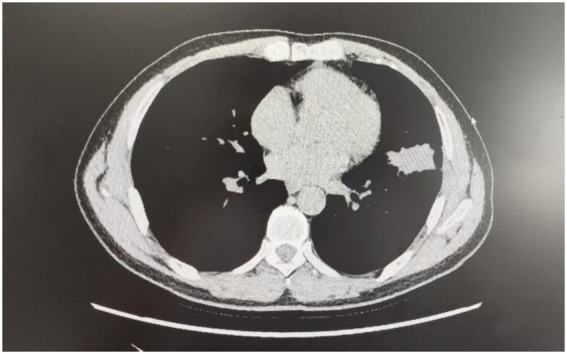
Pre-operative CT showed the dominant lesion in the left upper lobe, with an incidental nodule in the left lower lobe.
